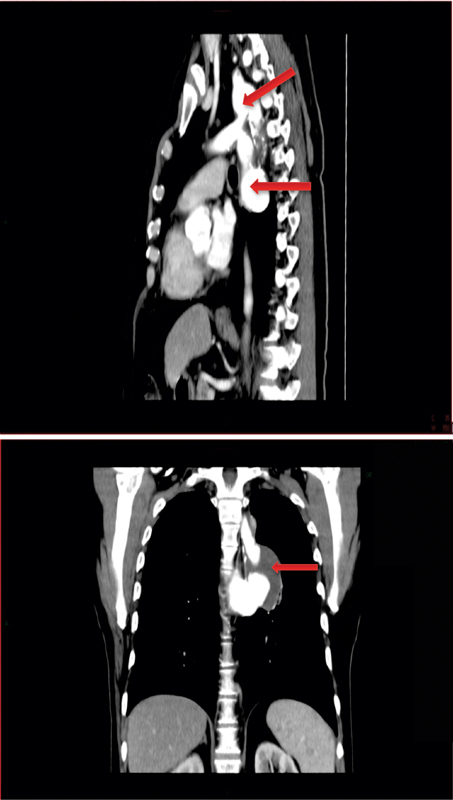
Computed tomographic findings. Upper panel, lateral view: proximal (upper arrow) and distal (lower arrow) large anastomotic pseudoaneurysms of the left subclavian to aorta bypass graft. Lower panel, frontal view: arrow points to large distal graft pseudoaneurysm.
